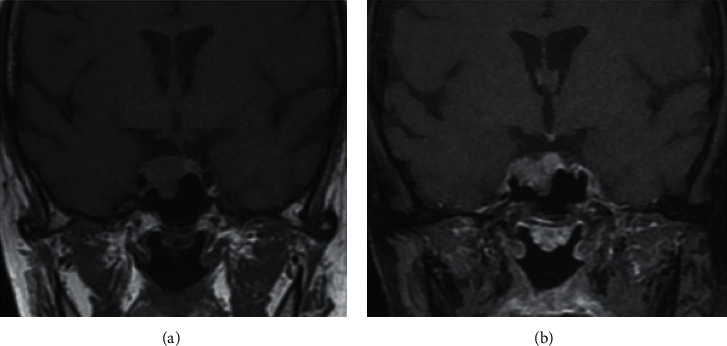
Pregadolinium (a) and postgadolinium (b) T1-weighted coronal images of pituitary MRI showing 17 mm adenoma with right cavernous sinus invasion and sellar floor erosion.
